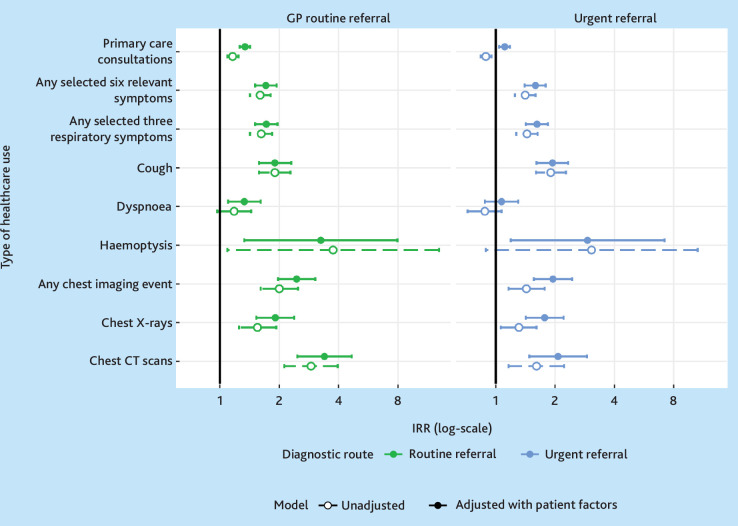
Unadjusted and adjusted Poisson model results showing the incidence rate ratios (IRRs, log-scale) for healthcare use by type 12 months to 1 month before diagnosis (with emergency diagnosed as the reference category). Log transformed data are shown for the economy of visualisation; exact values are included in Table 3. Any selected six symptoms include: appetite loss, weight loss, fatigue, cough, dyspnoea, and haemoptysis. Any selected three respiratory symptoms include: cough, dyspnoea, and haemoptysis. CT = computed tomography.
